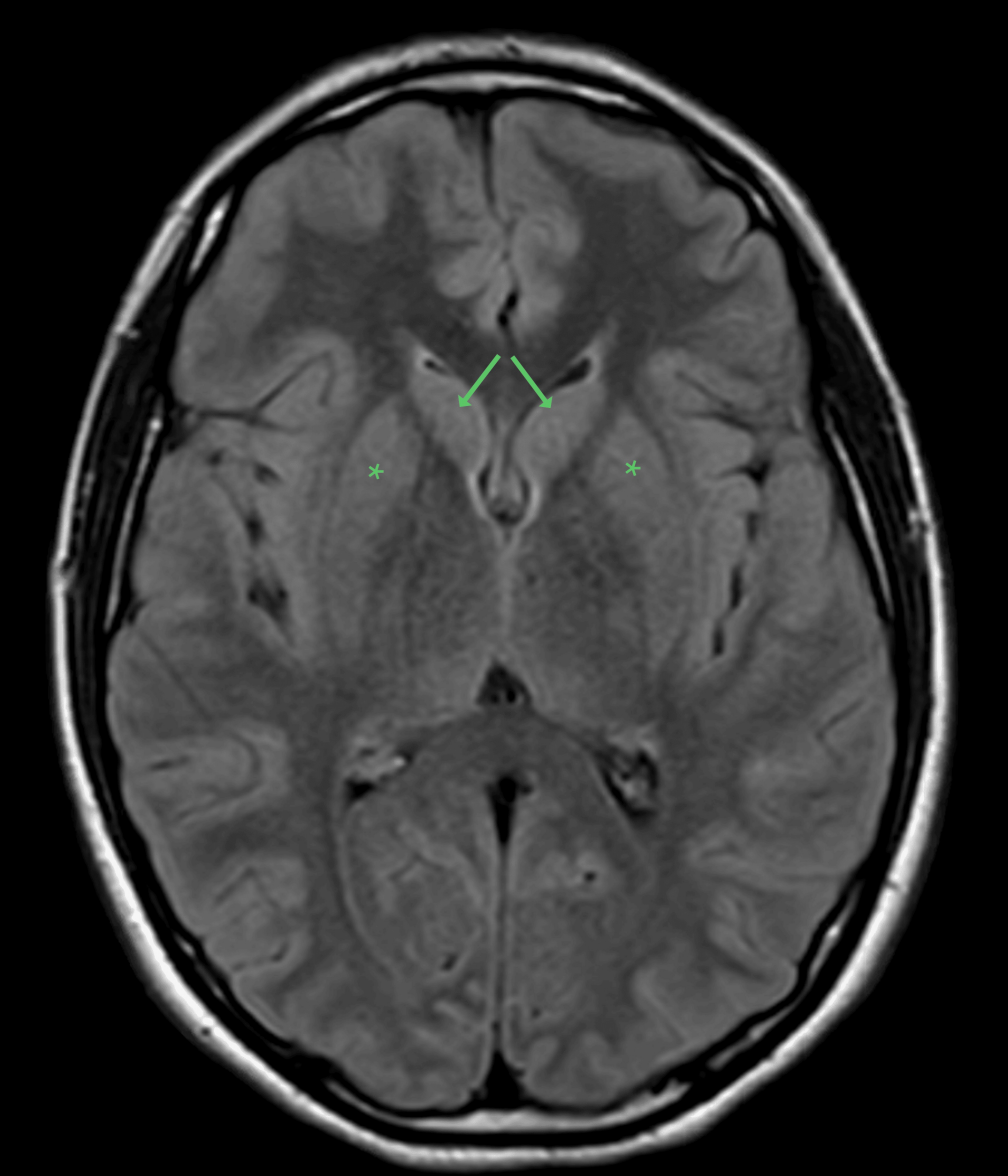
Axial MRI MRI Fluid Attenuation Inversion Recovery (FLAIR) image demonstrating enlarged caudate nuclei bilaterally (arrows) and mildly hyperintense bilateral putamina (stars) due to Sydenham's chorea.
